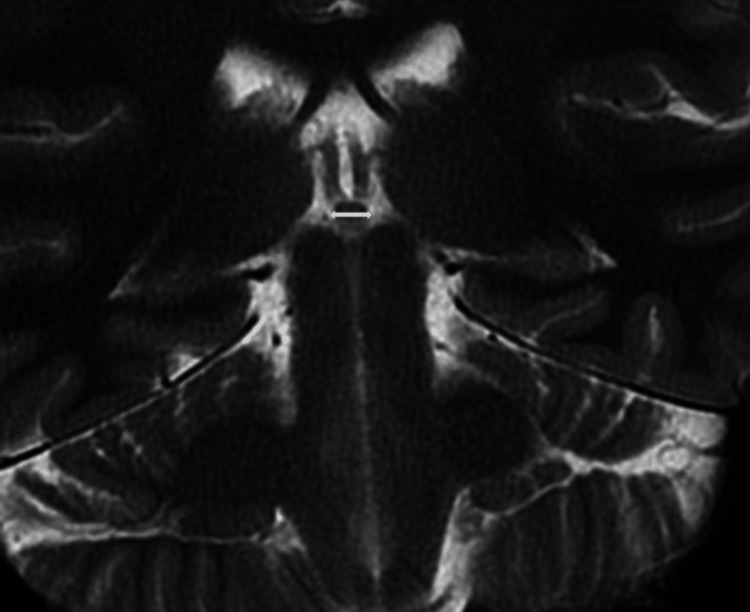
Coronal MRI images demonstrate the transverse length measurement of the pineal gland.
